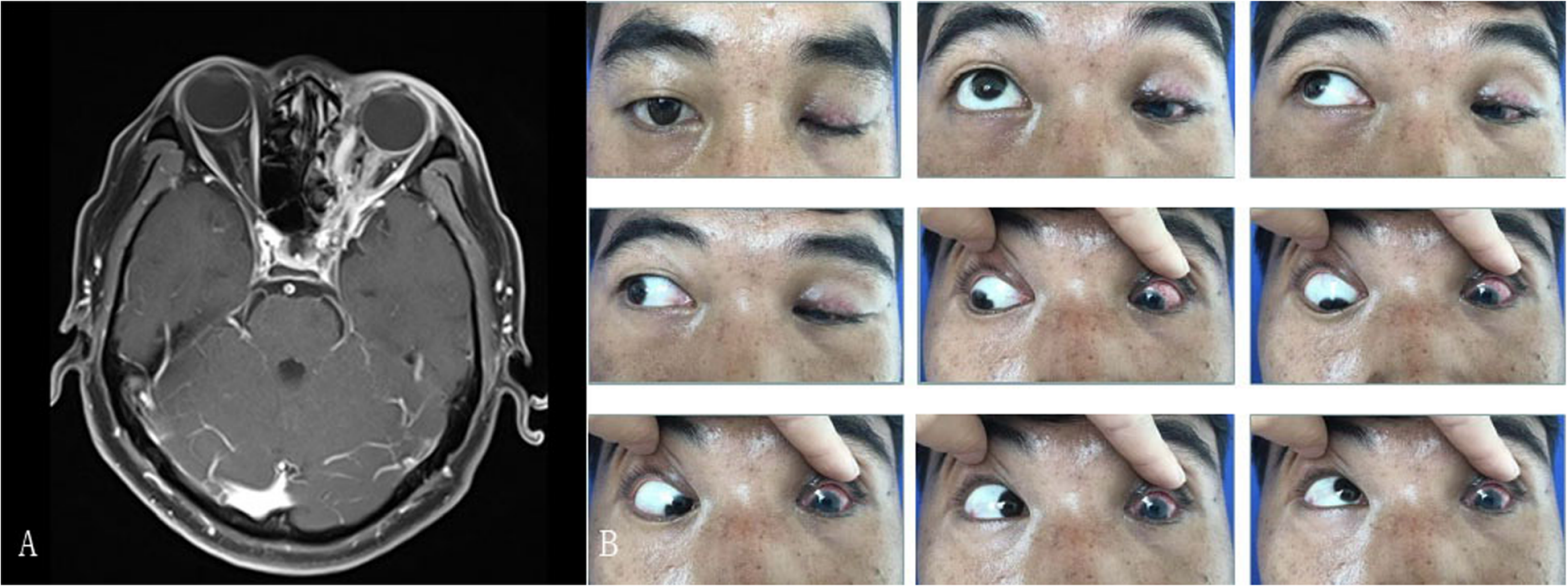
Orbital MRI scan and photographs. a MRI (enhanced) shows mild disordered structure of the left orbit. b Photographs show ptosis, ophthalmoplegia, and conjunctival congestion of the left eye
